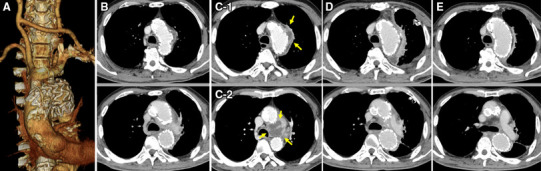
Contrasted computed tomography. a Three-dimensional image of a 2-debranch TEVAR. b Endograft infection was not demonstrated on admission. c-1 Contrast enhancement around the endograft and fluid collection inside the remnant aneurysm. c-2 Fluid filled cavity (roughly the size of a chicken egg) between aortic arch and pulmonary artery. d Postoperative 1 month. e Postoperative 2 years. No recurrence of endograft infection after thoracoscopic drainage and irrigation
